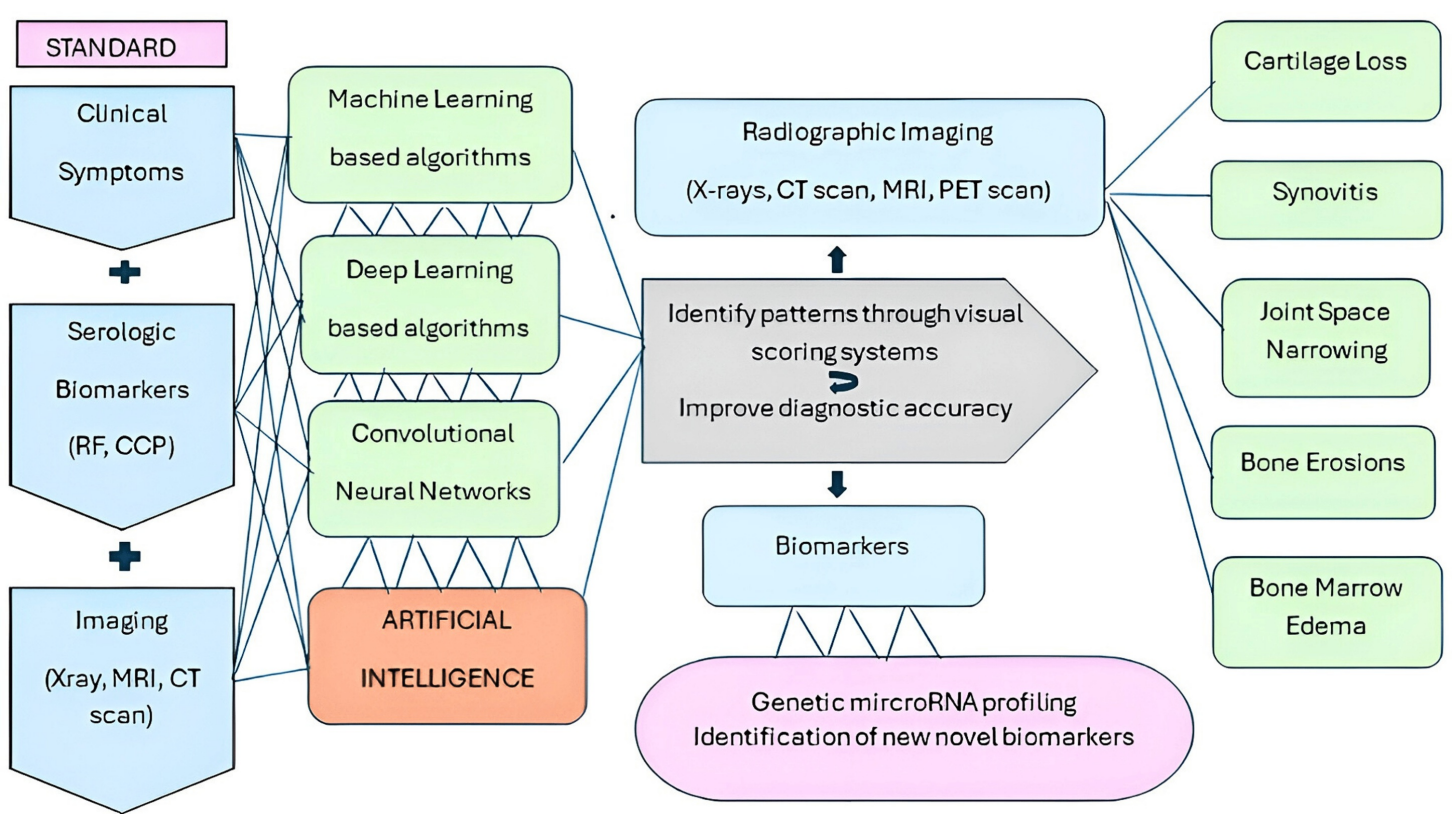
How AI improves the diagnostic modalities of rheumatoid arthritis compared to the standard protocol RF: rheumatoid factor; CCP: cyclic citrullinated peptide; MRI: magnetic resonance imaging; CT scan: computed tomography scan; PET scan: positron emission tomography
